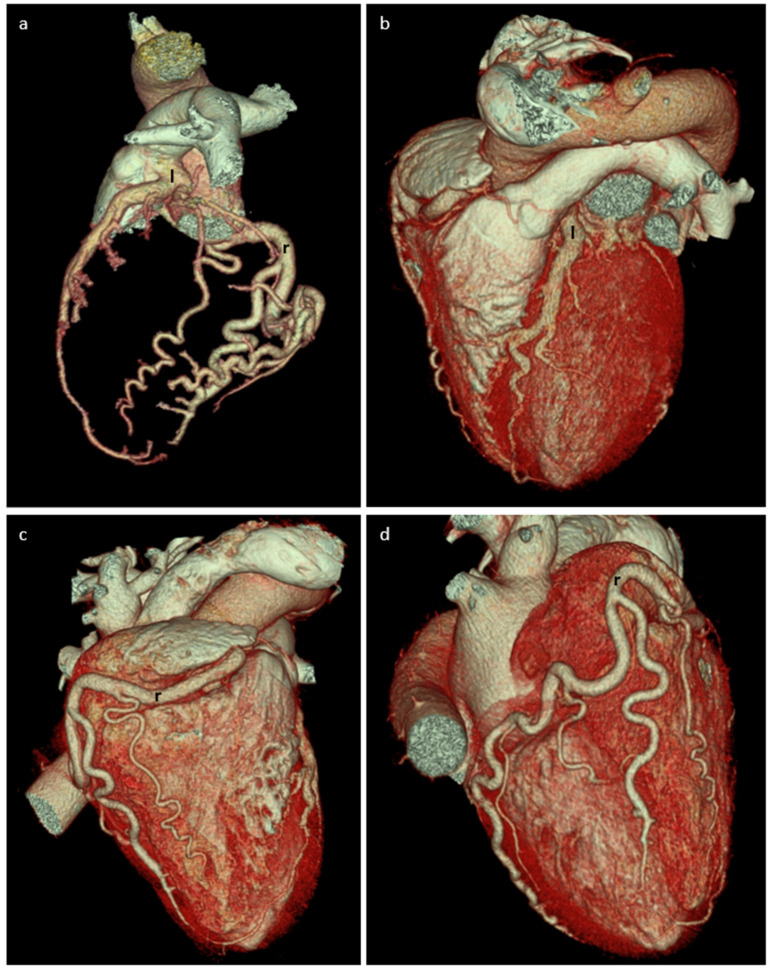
A patient with anomalous origin of the left coronary artery (l) from the pulmonary artery in computed tomography volume-rendering images (a,b), with associated dilation of the right coronary artery (r) (c,d).
